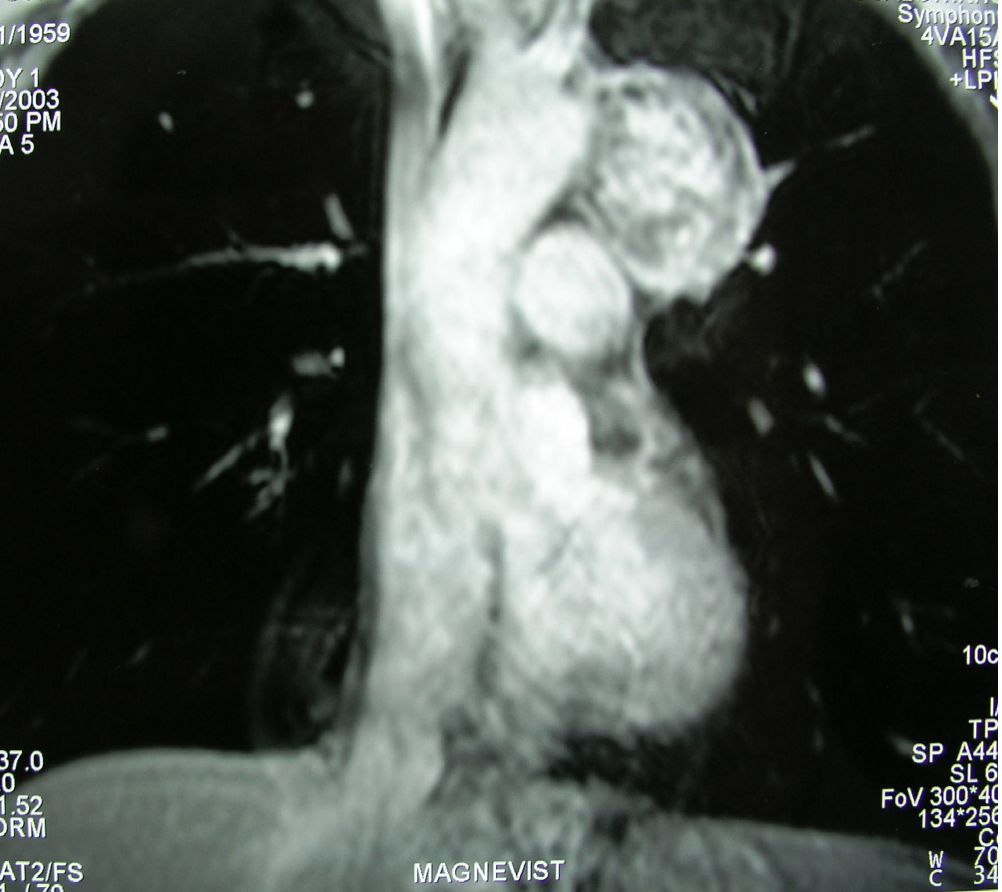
MRI of the chest showing an encapsulated mass in theaortopulmonary window, suggesting a benign lesion.
